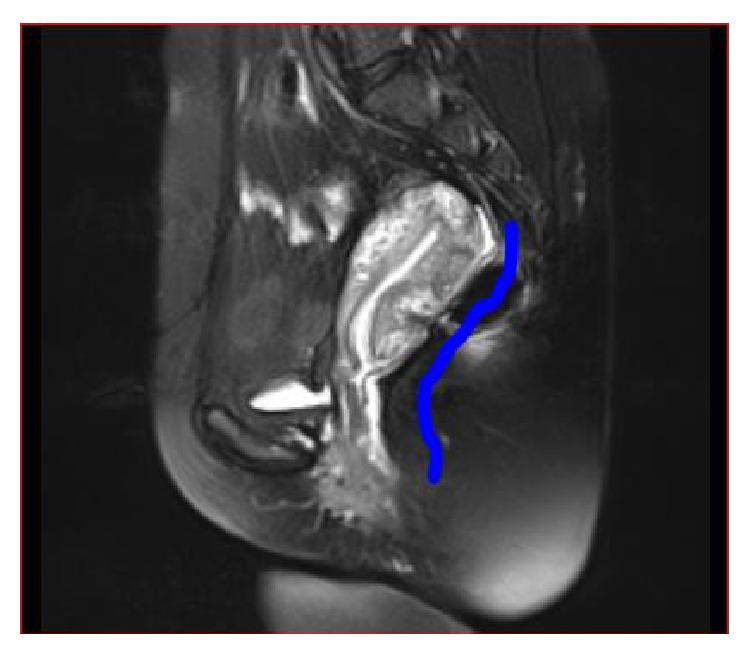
MRI appearance of the pelvis with uterus at 12 months postpartum.
